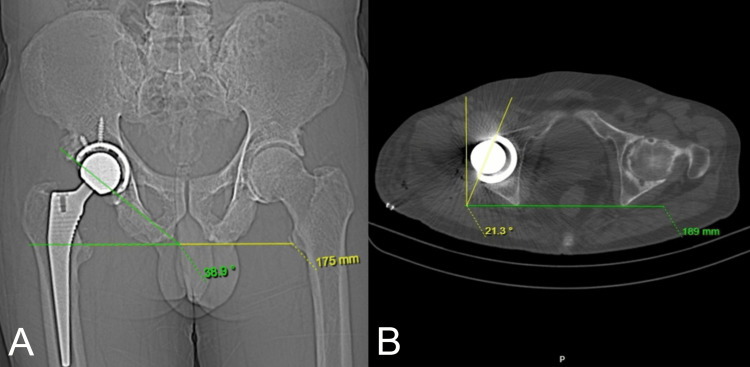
CT scan used to measure acetabular component positioning (A) Coronal section showing acetabular component inclination (B) Axial section showing acetabular component anteversion
